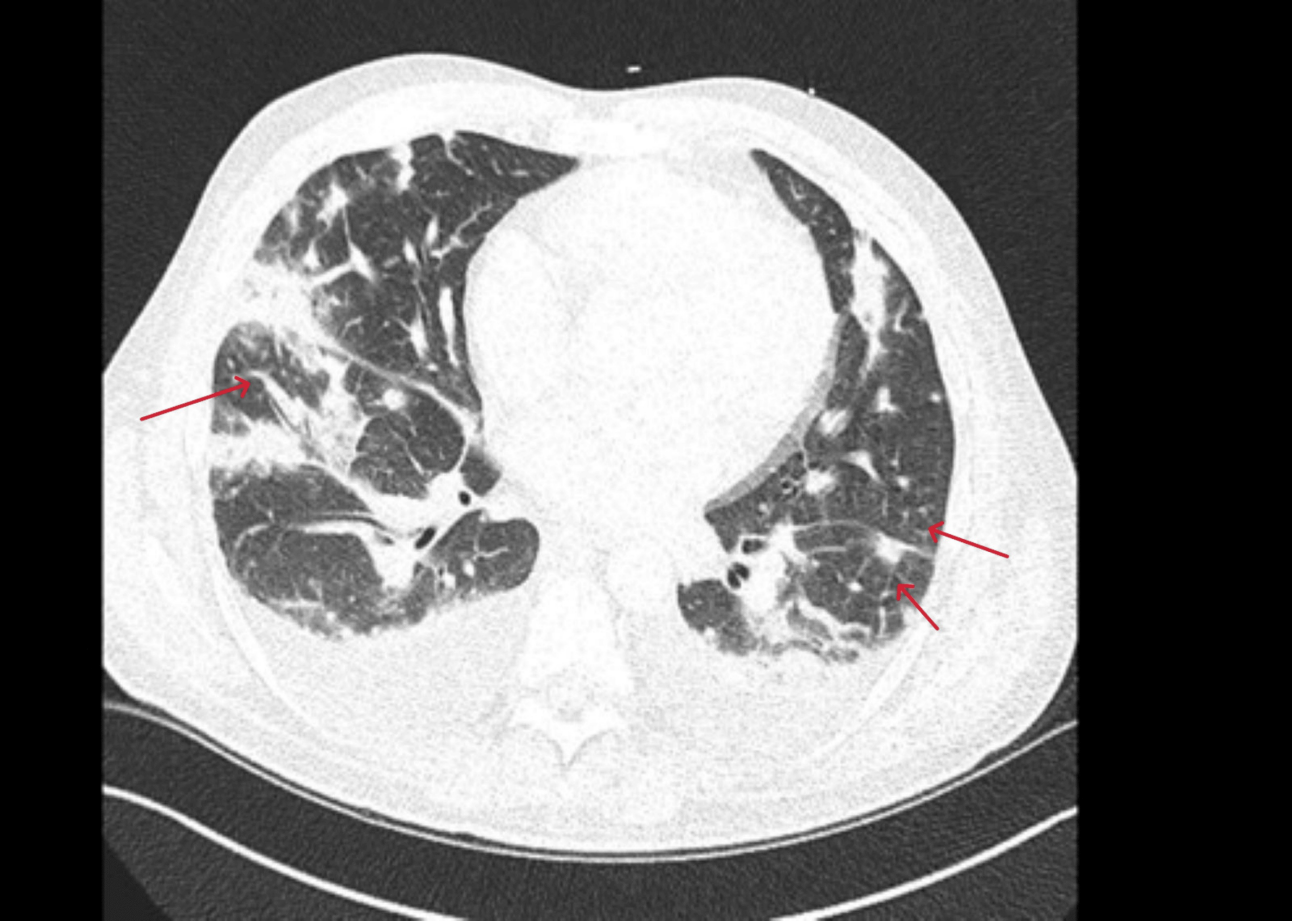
Axial computed tomography angiography of the chest demonstrating multiple bilateral peripheral cavitary pulmonary nodules (arrows), consistent with septic pulmonary emboli in the setting of methicillin-resistant Staphylococcus aureus bacteremia.
